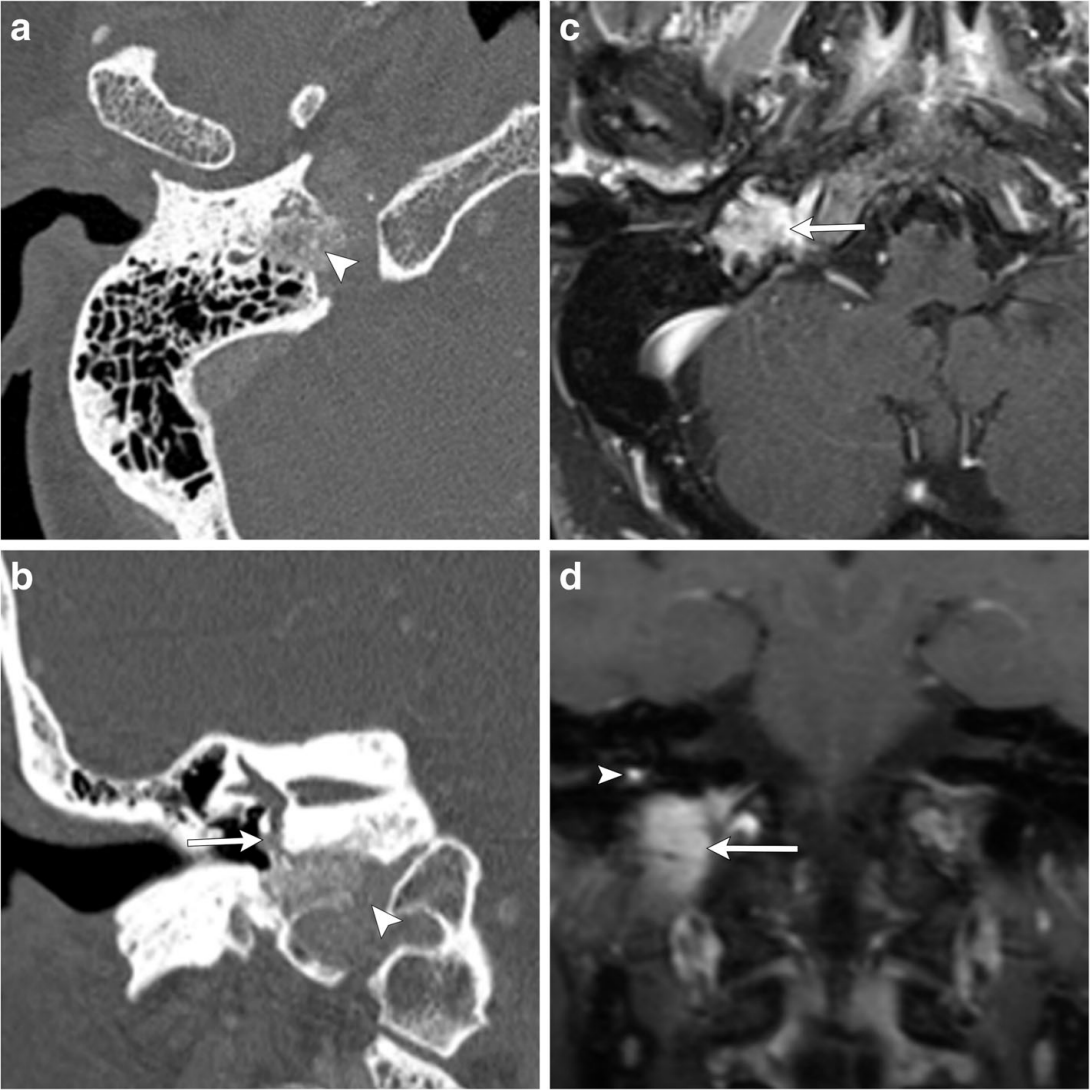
Axial (a) and coronal (b) CT images demonstrate erosion of the right jugular bulb, with a moth-eaten appearance of the walls of the right jugular foramen (white arrowheads). This destructive lesion expands into the middle ear, with invasion of the cochlear promontory (white arrow). Axial (c) and coronal (d) T1-weighted post-contrast MR images demonstrate avid enhancement of the mass which arises between the jugular foramen (white arrow) and the cochlear promontory (white arrowhead). The mass fills the jugular foramen and extends into the middle ear anterolaterally
